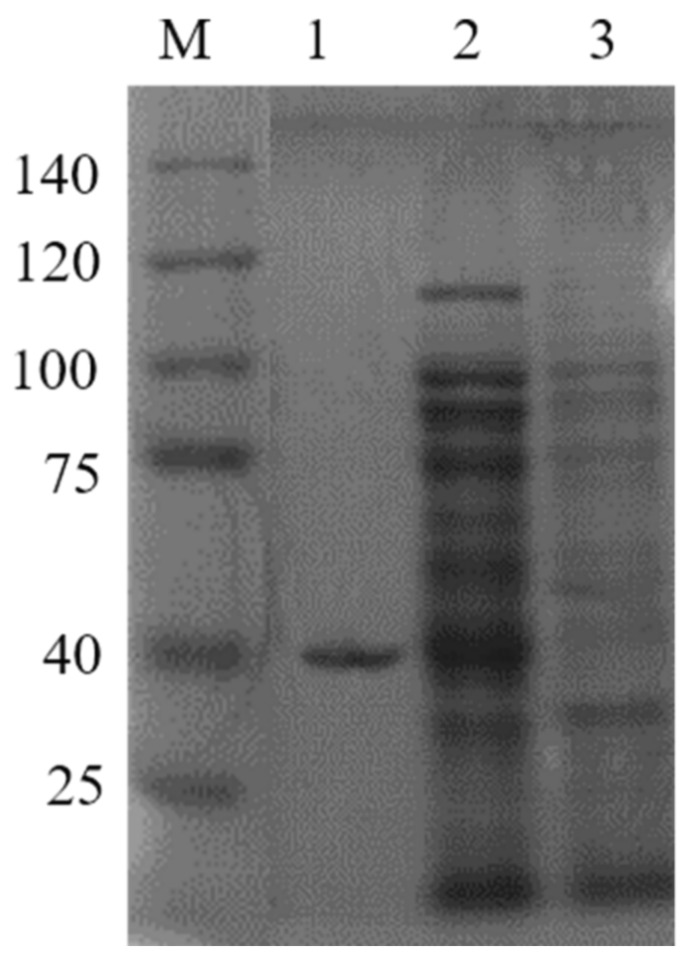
SDS-PAGE analysis of prokaryotic expressed and purified GST-CaM fusion proteins. Line 1: purified GST-CaM fusion protein; line 2: ultrasound supernatant of GST-CaM/BL21 (DE3); and line 3: ultrasound precipitation of GST-CaM/BL21 (DE3).
